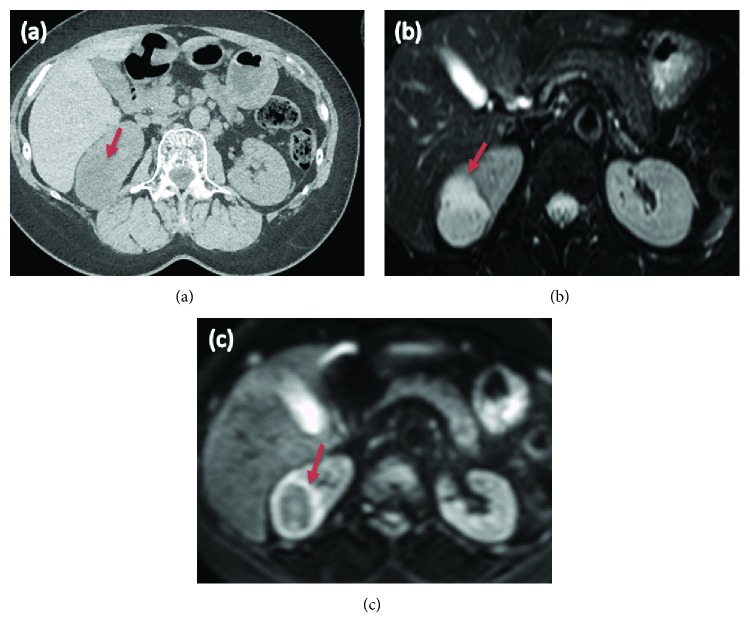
(a) CT scan demonstrated a cyst in the upper half of the right kidney and no septa or calcifications (arrow). (b) T2-weighted MRI showed a cystic tumor with a high signal (arrow). (c) Diffusion-weighted MRI (DW-MRI) showed rim enhancement (arrow).
